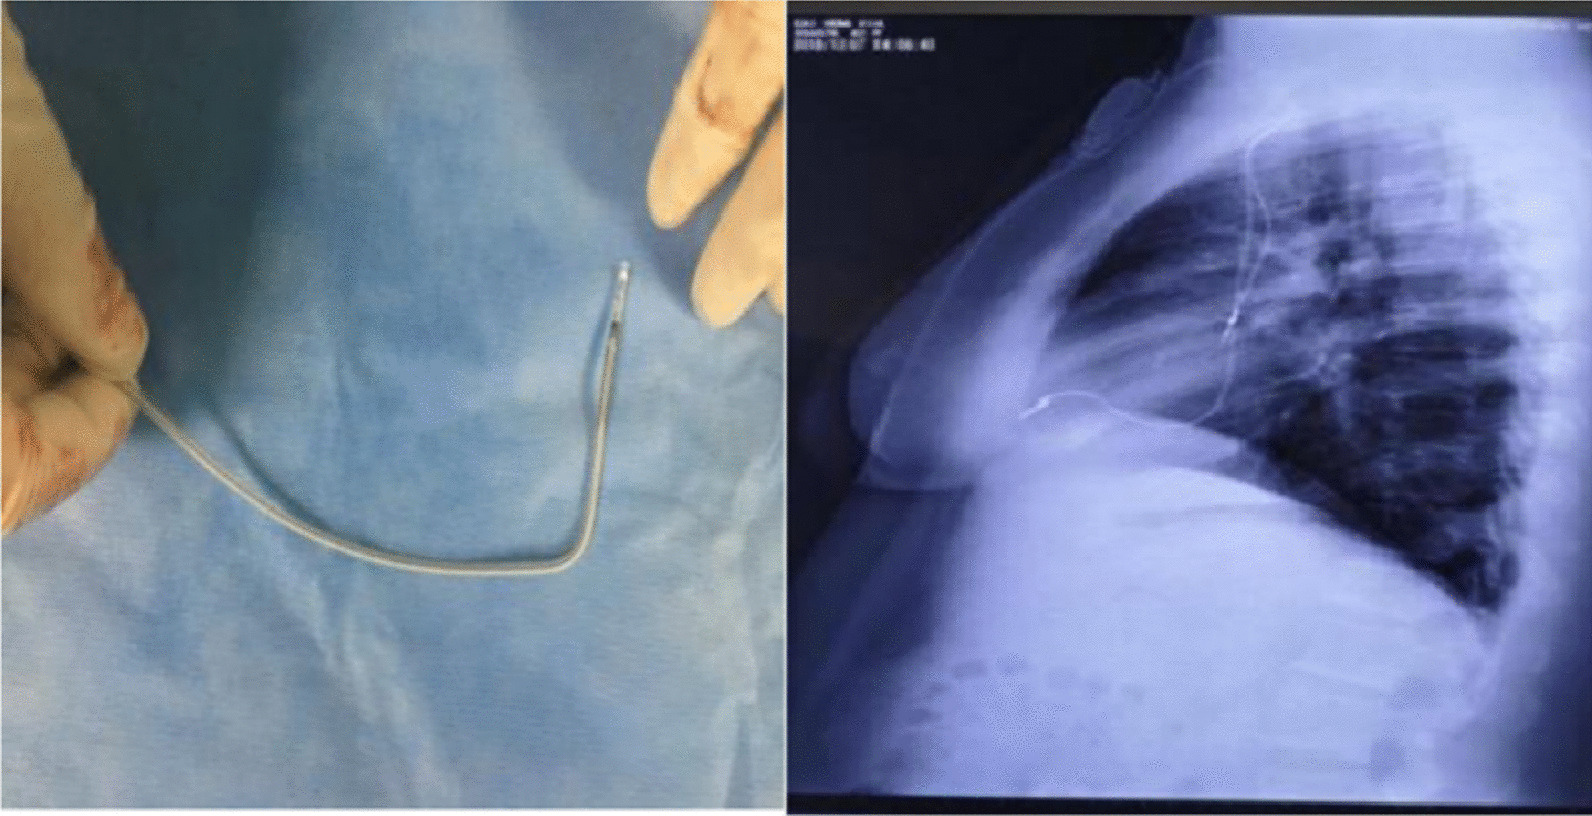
Atrial lead with a bigger bend before implantation (left). X-ray shows the two positions of leads 5 months after cesarean section (right)
